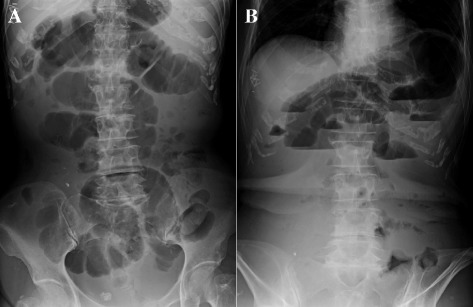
Abdominal X-ray before surgery. Upright position (A), supine position (B).
